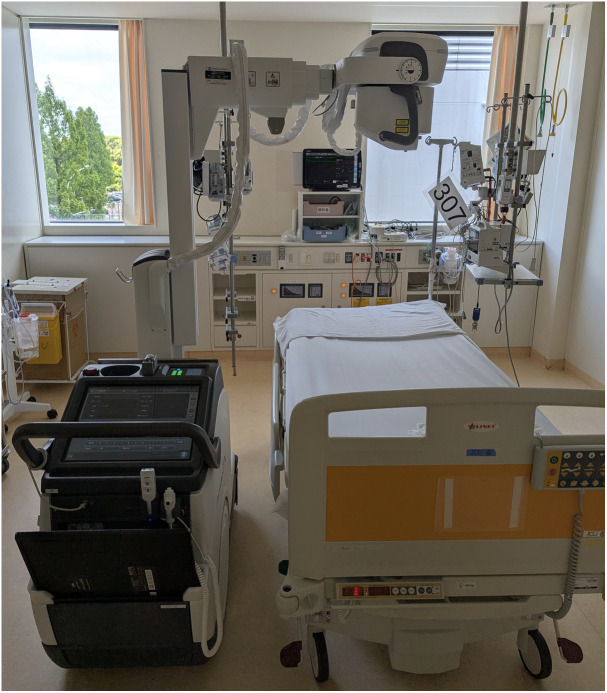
Portable dynamic digital radiography (DDR) system used for bedside imaging in ICU patients. The portable DDR system used in the present study for bedside assessment of respiratory motion in mechanically ventilated ICU patients. The system enables sequential low-dose chest radiographic imaging during tidal breathing and allows dynamic visualization of respiratory-related changes in lung motion and lung area at the bedside.
